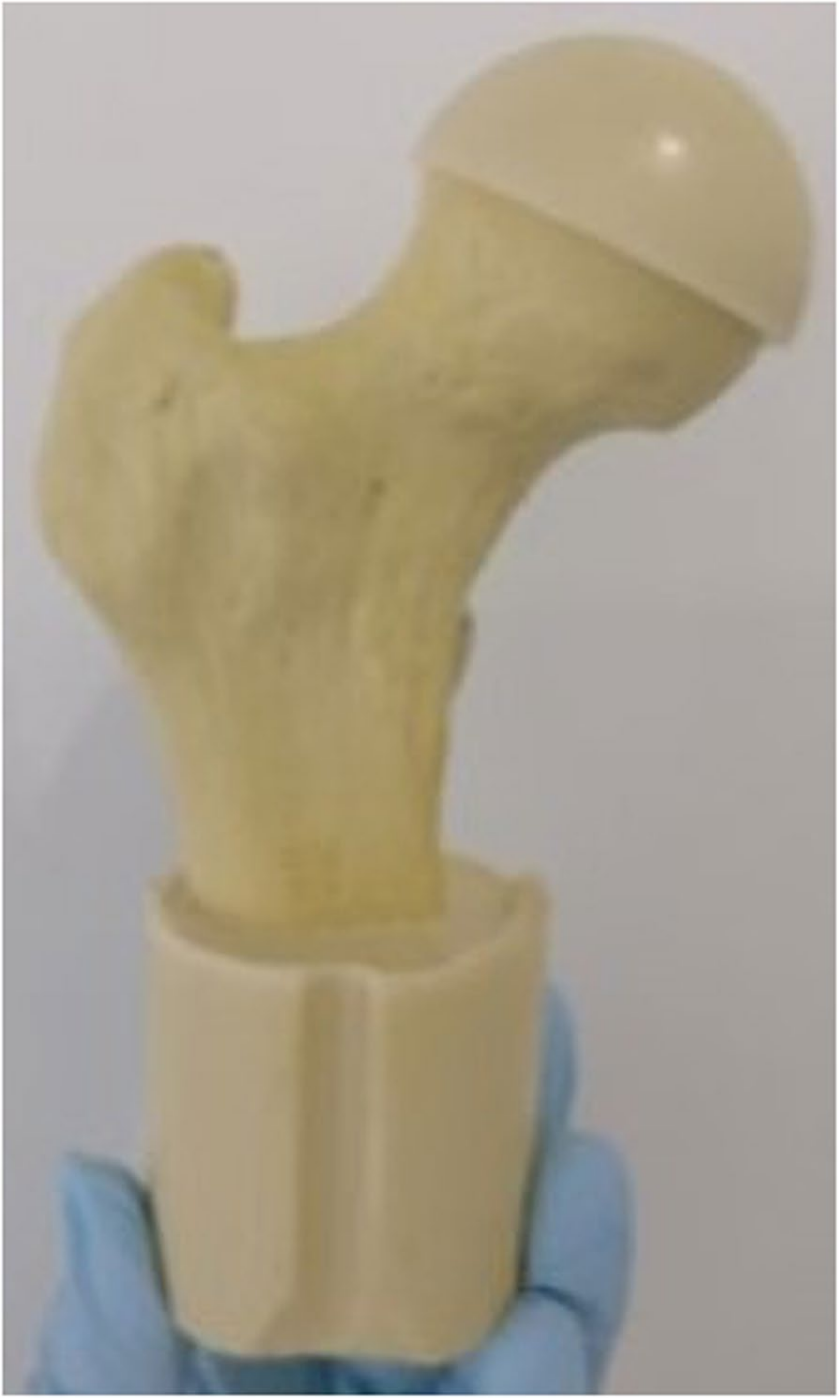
Embedded truncated generic Femur in preparation for μ-CT scanning
